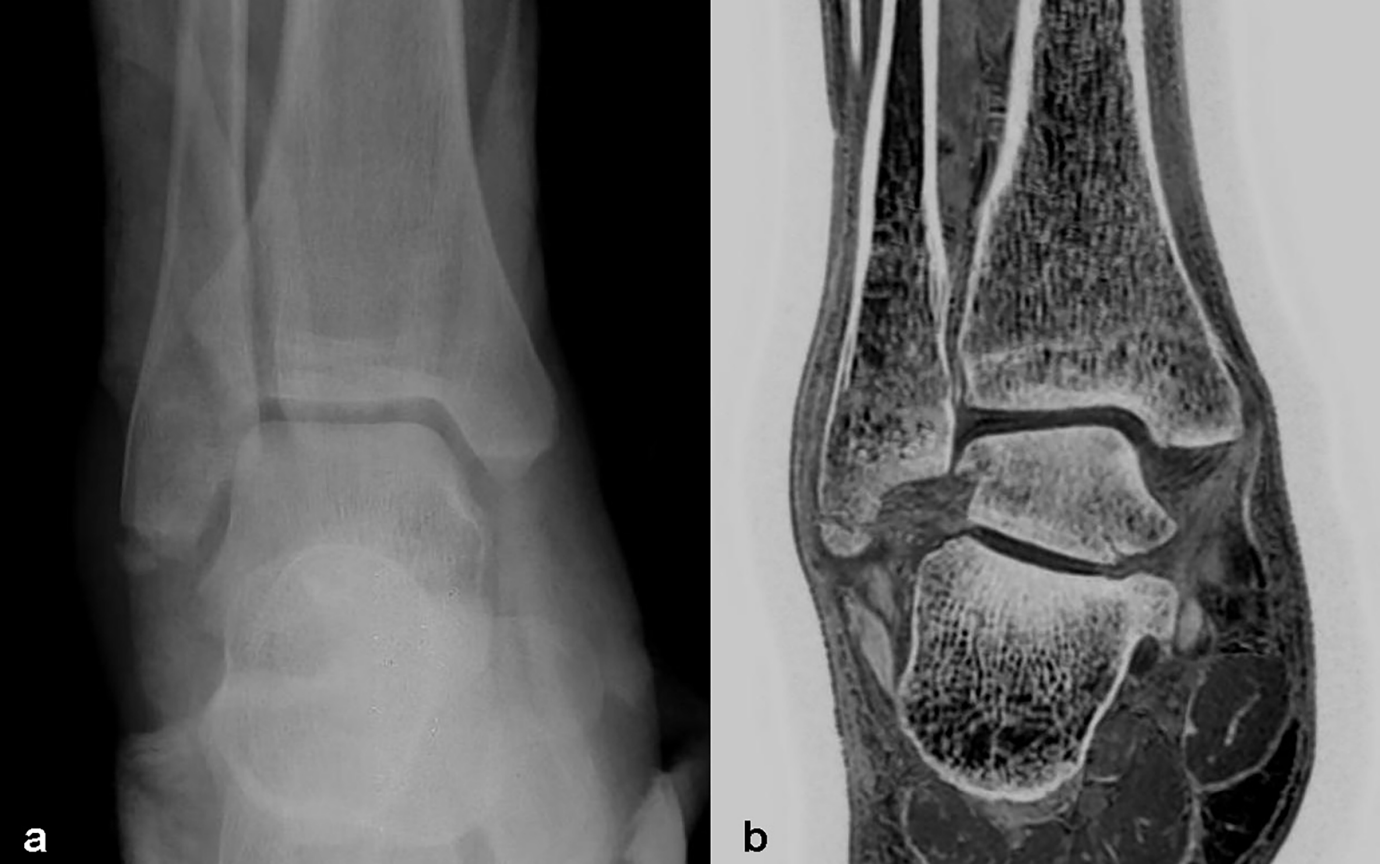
Images in a 40-year-old male with right distal fibula fracture. (A, B) were positive X-Ray and coronal FRACTURE images. Fracture lines and bone fragments were consistent in X-Ray and FRACTURE images.
